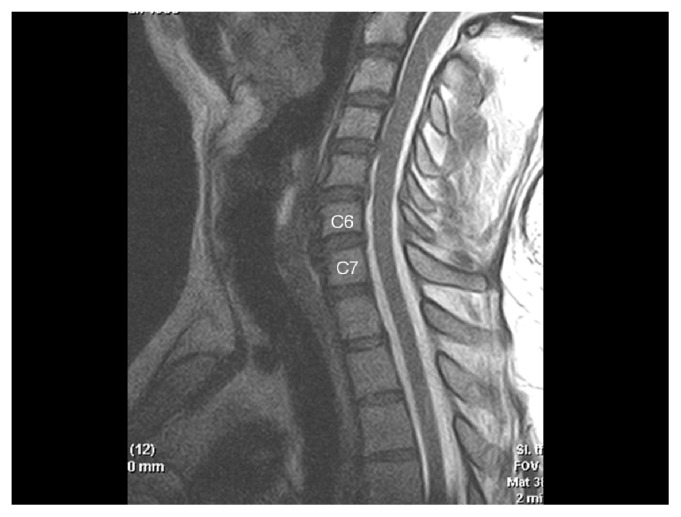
Sagittal T2-w MRI showing compression on the esophagus caused by anterior osteophytes.
